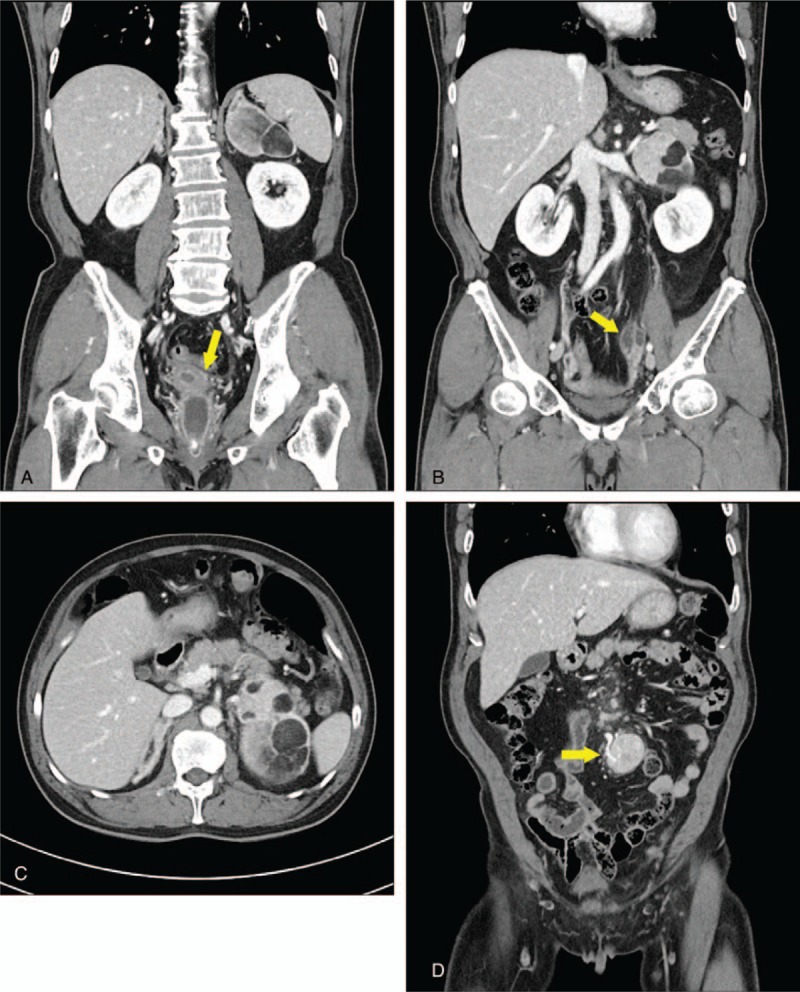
Abdominal computed tomography showing (A) Mullerian structures with fluid collection in the vaginal cavity (arrow); (B) Dilated, thickened left fallopian tube compatible with salpingitis (arrow); (C) A 10 cm myelolipoma in the left adrenal gland; (D) A 3.2 cm hypervascular mass in the small bowel mesentery (arrow).
